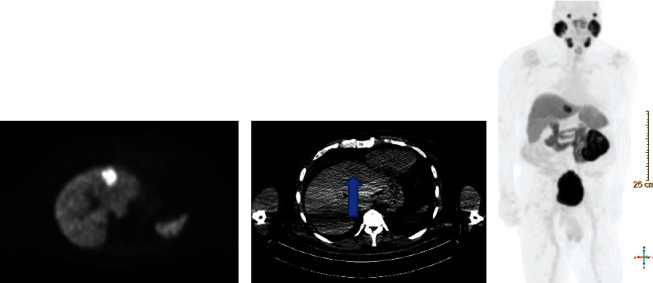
(a–c) PET-CT with 18F-PSMA. Axial (a) and 3D (c) PET images demonstrate a large focus of markedly and heterogeneously increased radiotracer uptake in the left lobe of the liver. Unenhanced low-dose CT image at the corresponding level with narrow window demonstrates a subtle hypodense ill-defined nodule anteriorly in the left hepatic lobe (arrow).
